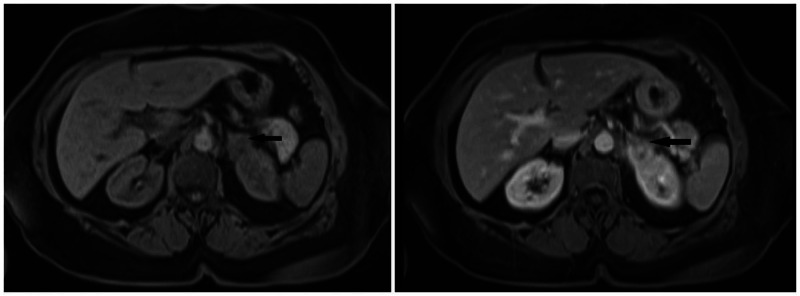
MRI of abdomen W/O and W/contrast showing left suprarenal mass inseparable from the upper pole of the left kidney and left adrenal gland (arrow) with T1 and T2 slightly hypointense signal compared to renal cortex, restricted diffusion and postcontrast enhancement.
